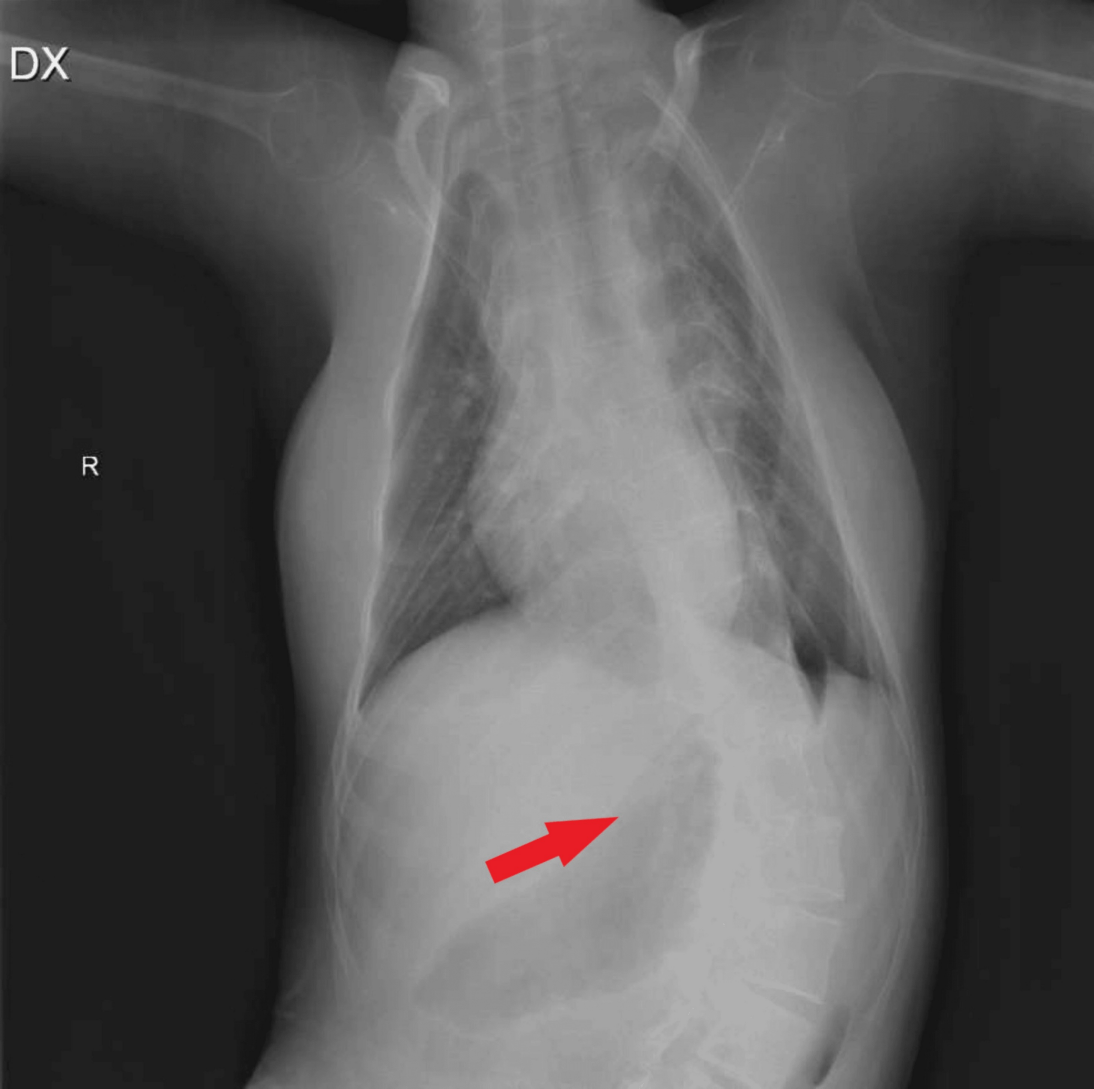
Preoperative chest X-ray of the patient shows severe scoliosis.
